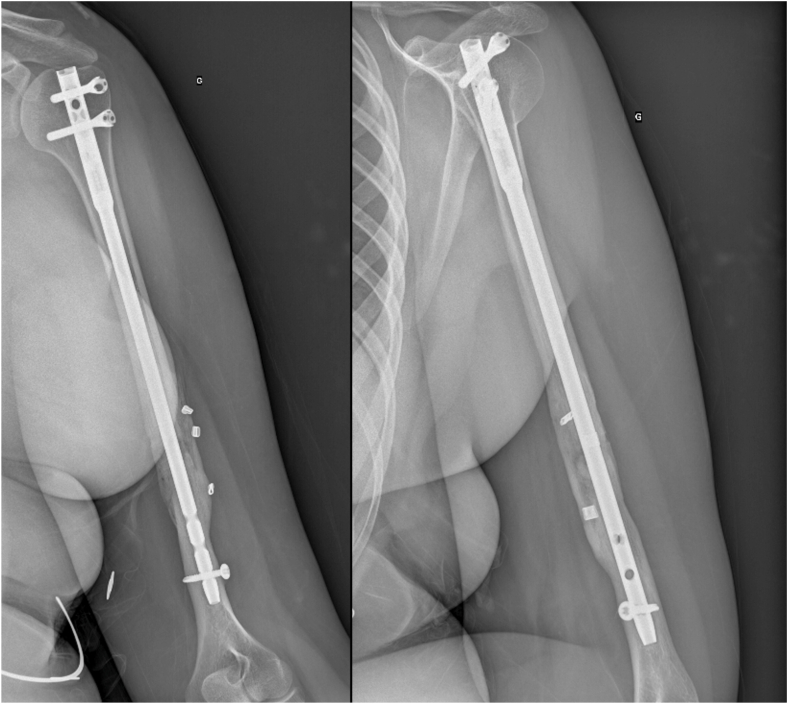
Anteroposterior and lateral radiographs at 7 months post-operatively demonstrate fracture union and graft incorporation.
